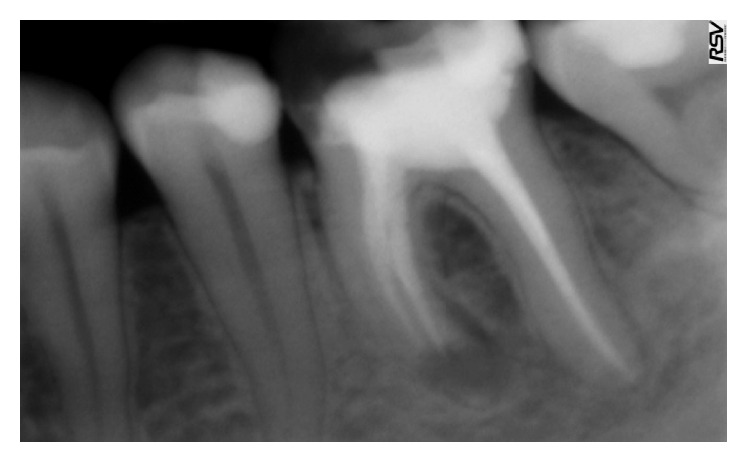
Radiograph at 2-month follow-up.
